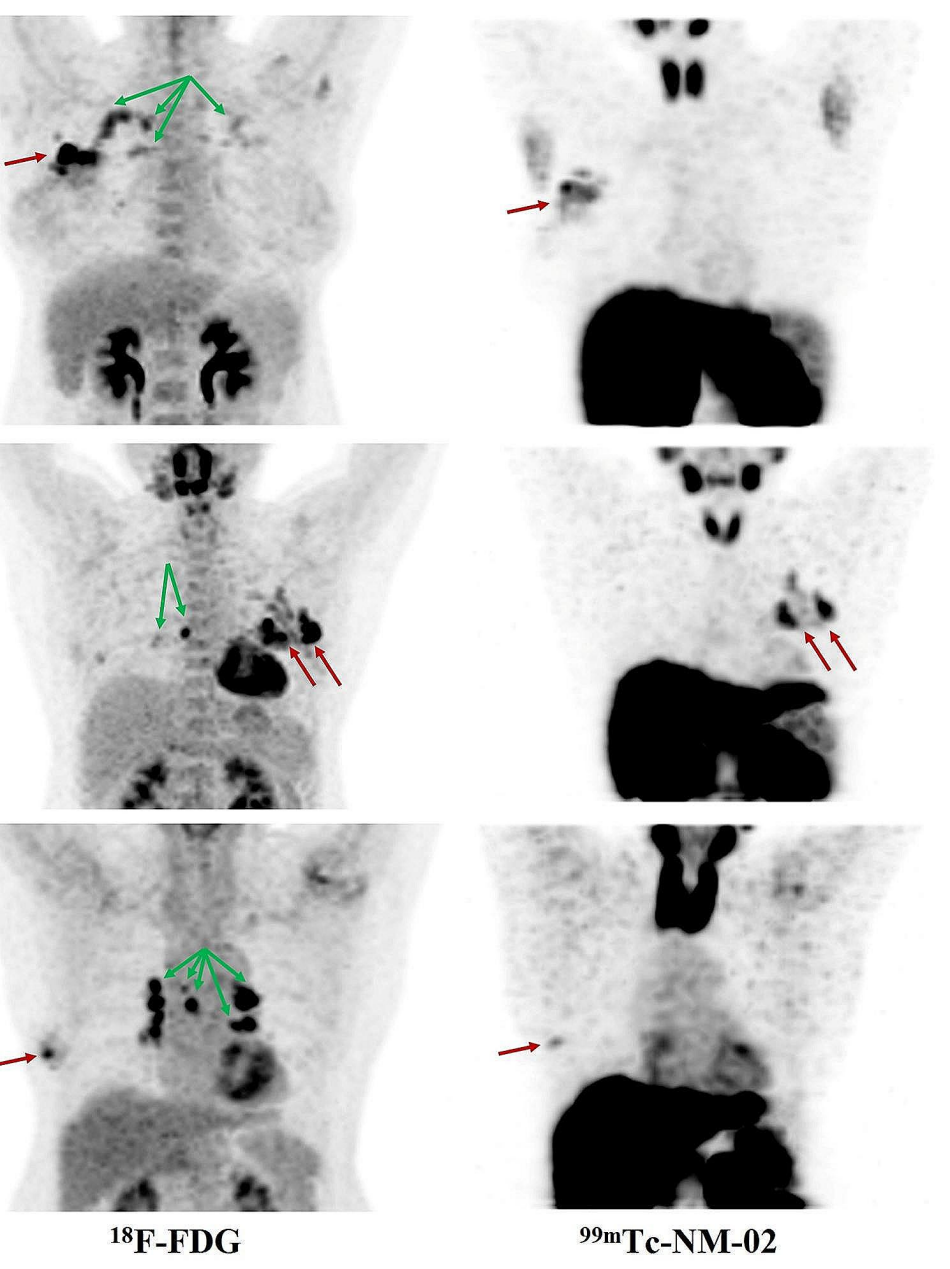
^18^F-FDG PET/CT and ^99m^Tc-NM-02 SPECT/CT imaging of three patients with breast cancer (red arrows) and chest inflammation (green arrows) at 1 h post-injection
